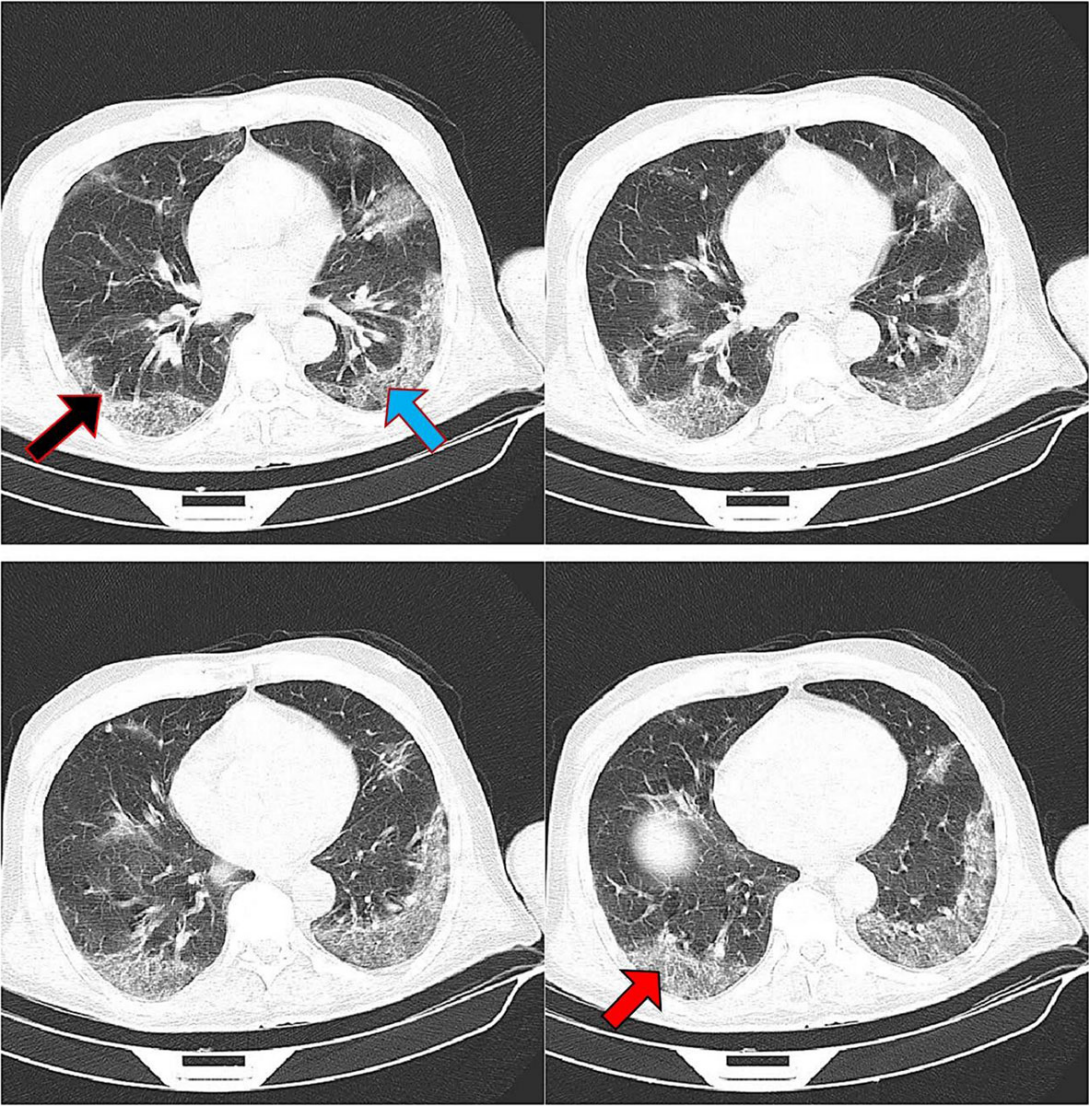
A 77-year-old male patient presented with fever, chills, and dry cough, who was admitted to the regular ward and was discharged on day 10 of admission. Peripheral multilobar ground glass opacities (red arrow), parenchymal bands (black arrow), and crazy paving (blue arrow) are observed in the CT scan. The total CT severity score was calculated as 15, and the disease severity was moderate
